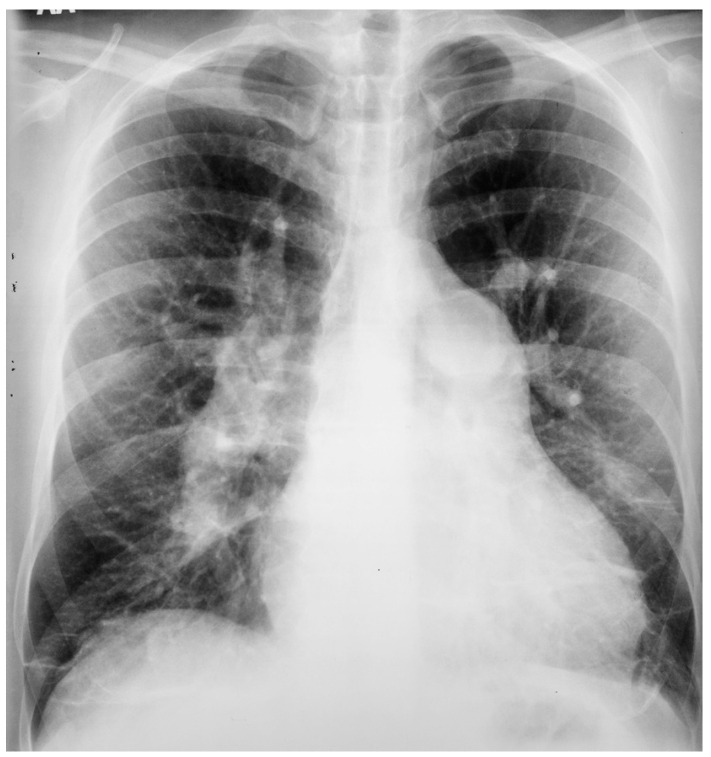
Posteroanterior chest X-ray of ostium secundum atrial septal defect (OSASD): This is a chest X-ray of a 46-year-old male with an uncorrected OSASD. The image is slightly rotated. There is a good inspiration. The cardiothoracic ratio is increased. The pulmonary trunk is enlarged and there is a lateral rim of calcification. Compared to the enlarged central pulmonary circulation the more peripheral pulmonary vessels are much smaller (called pruning), suggesting pulmonary vascular obstructive disease. The lower right heart border demonstrates right atrial enlargement. The aortic knob is smaller than the pulmonary trunk, indicating a significant left to right shunt.
